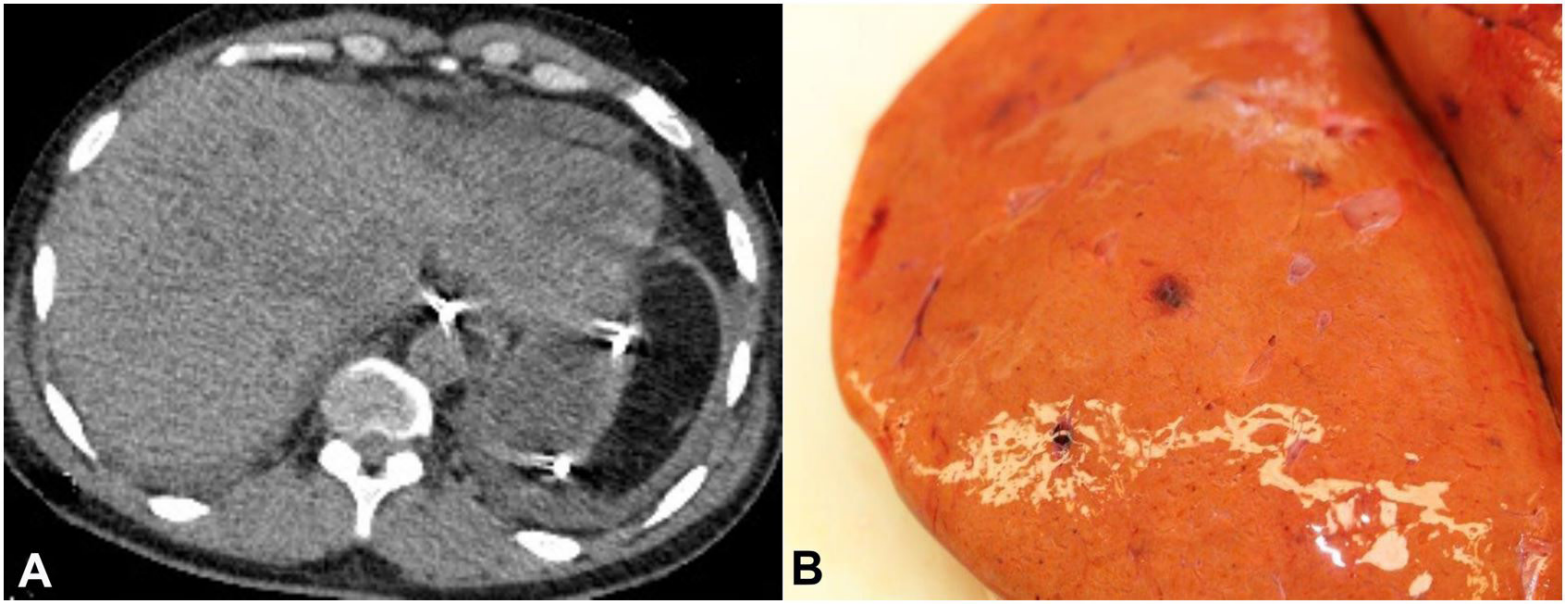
A – Antemortem CT scan of the abdomen showed innumerable low attenuation lesions throughout the liver, B – At autopsy, multiple hemorrhagic foci were observed throughout the liver.
